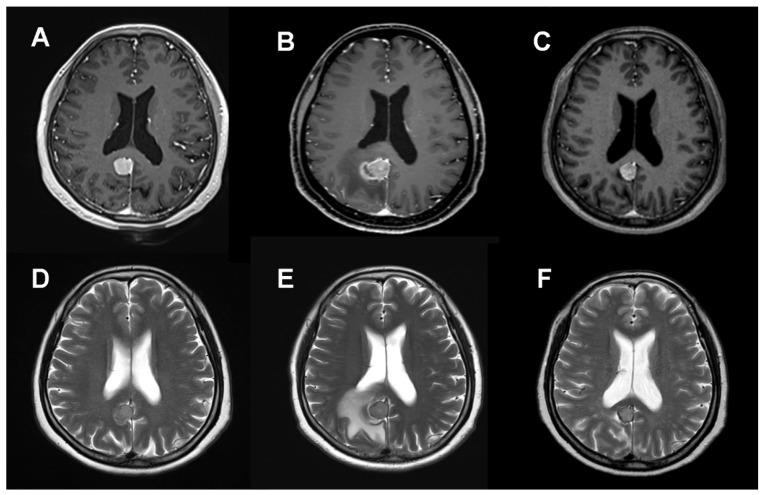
Axial contrast-enhanced T1- and T2-weighted magnetic resonance imaging scans of meningioma at the posterior falx at the time of radiosurgery (A,D), 6 months later (B,E), and 3 years later (C,F). The 6-month follow-up images demonstrate peritumoral edema around the treated meningioma, which was managed with high-dose steroids. Meningioma and peritumoral edema were stabilized 3 years after radiosurgery. Radiosurgery was performed using a tumor margin dose of 15 Gy.
